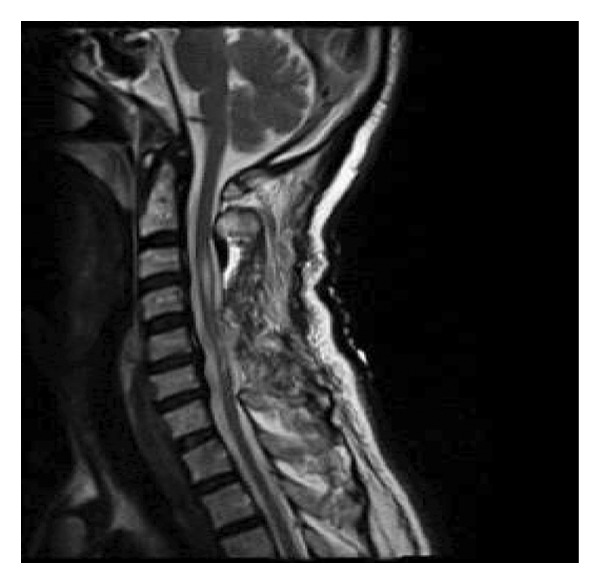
Postoperative T2‐W MRI: hyperintense signal change is seen at C3/C4 level.
